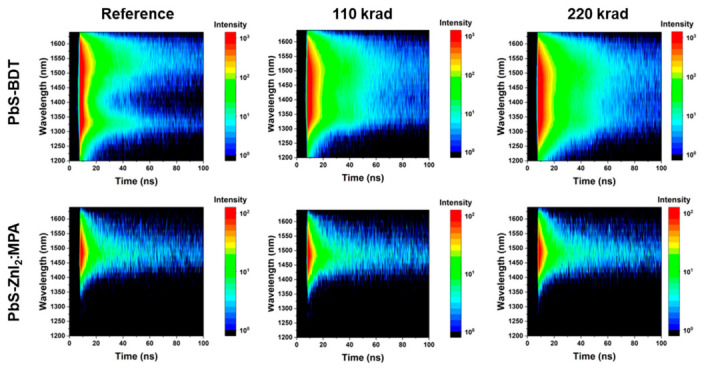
Time-resolved photoluminescence (TR-PL) contour map of CQD layers as a function of ligand type and X-ray irradiation dose, comparing PbS-BDT and PbS-ZnI_2_:MPA from non-irradiated conditions to 220 krad.
